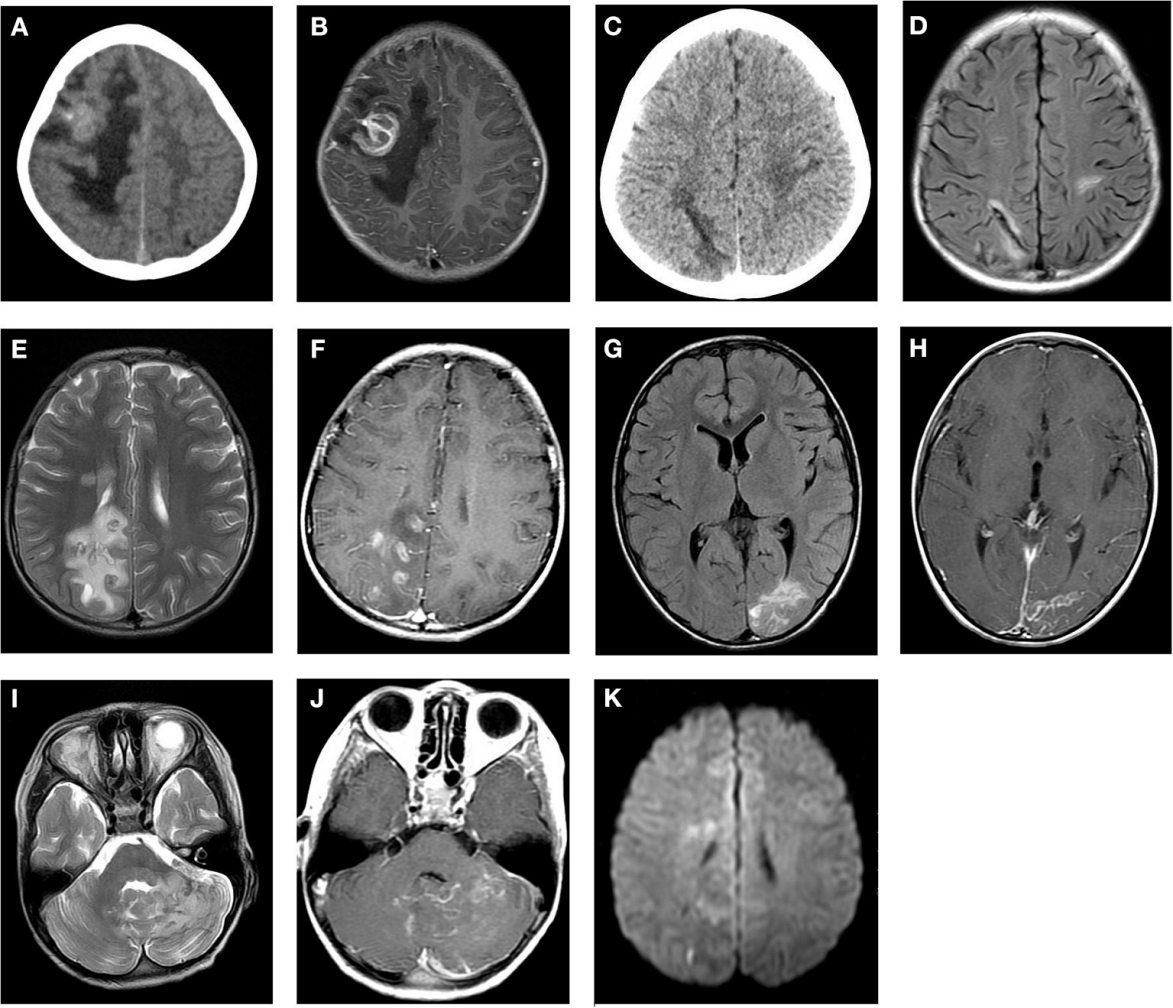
Cranial CT and MRI features of the patients with cerebral paragonimiasis are shown. CT images showed the lesion in the right frontotemporal lobe, which is a mass of equal or slightly high density, surrounded by patchy low-density edema (A). The T1WI enhancement scans showed that the lesion was a ring-like enhancement, surrounded by edema (B). CT images showed a strip-like low-density lesion in the right parietal lobe surrounded by mild edema (C). On the T2/FLAIR images, the lesion was a strip-like low signal surrounded by a high signal, which is the classic “tunnel sign” (D). On the T2WI scans, the lesion in the right parietal occipital lobe was nodular and a circular low or slightly high signal, accompanied by peripheral patchy edema (E). The lesions showed obvious nodular and circular enhancement on the T1WI enhancement scans (F). On the T2/FLAIR images, the left occipital lobe lesions were strip-like and sheet with slightly high signals, and the peripheral edema was not obvious (G). On the T1WI enhancement scans, the lesion showed strip enhancement (H). On the T2WI scans, the left cerebellar hemisphere showed an uneven increased signal, which had nodular and striped mixed signals with unclear boundaries (I). On the T1WI scans, the lesions showed ring-like and strip-like enhancements, and the adjacent meninges were thickened and significantly enhanced (J). On the DWI scans, an equal or slightly high signal is shown (K).
